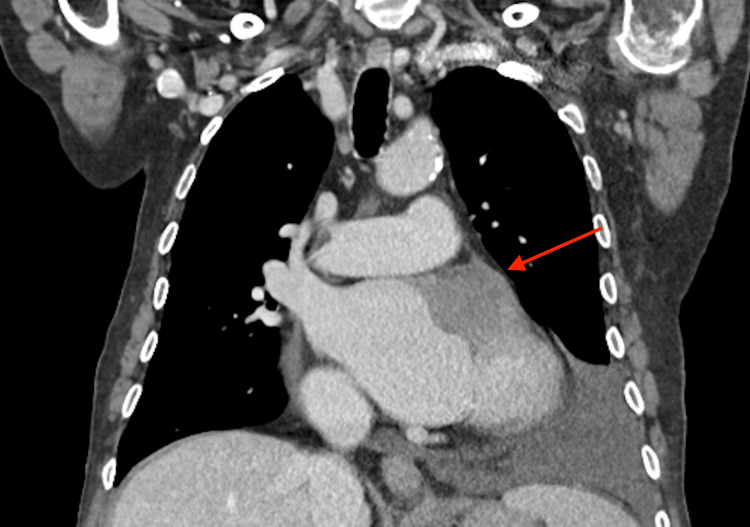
Coronal section of CT-thorax demonstrating hypodensity at the level of the left auricle: left pleural effusion
